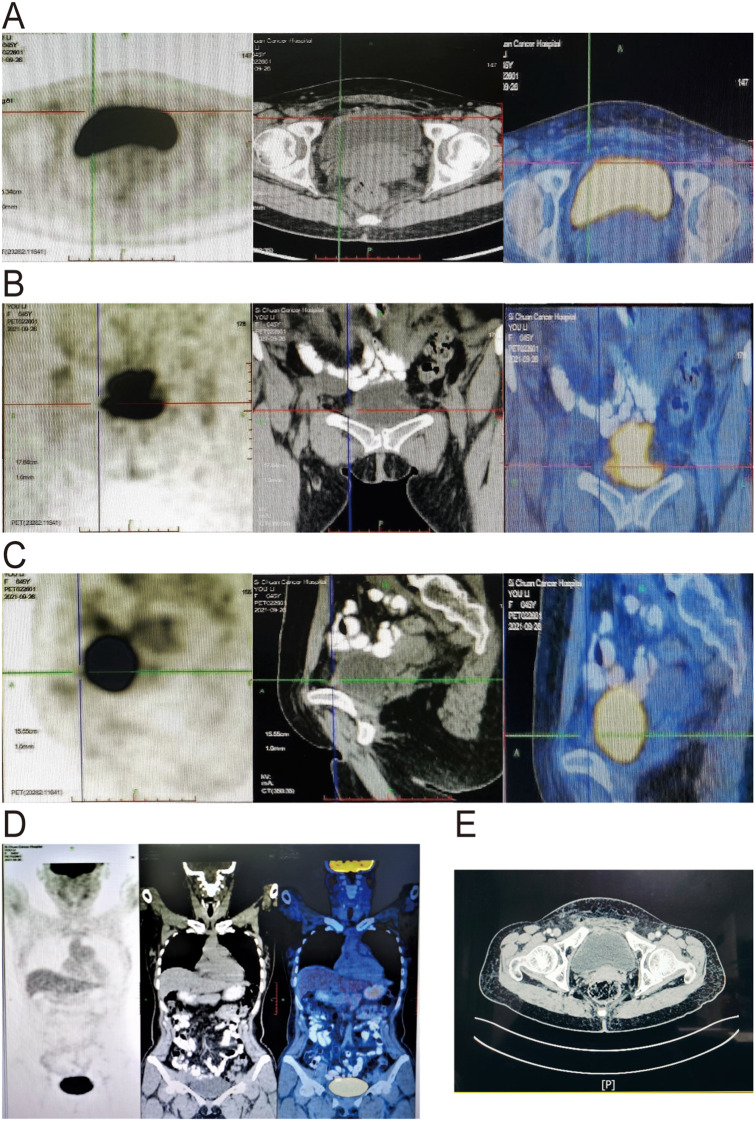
Follow-up images. (A-D) PET-CT showed postoperative changes with a slightly thickened anterior bladder wall predominantly on the right side, without significant metabolism. (E) CT showed postoperative changes with a slight thickening of the anterior bladder wall.
